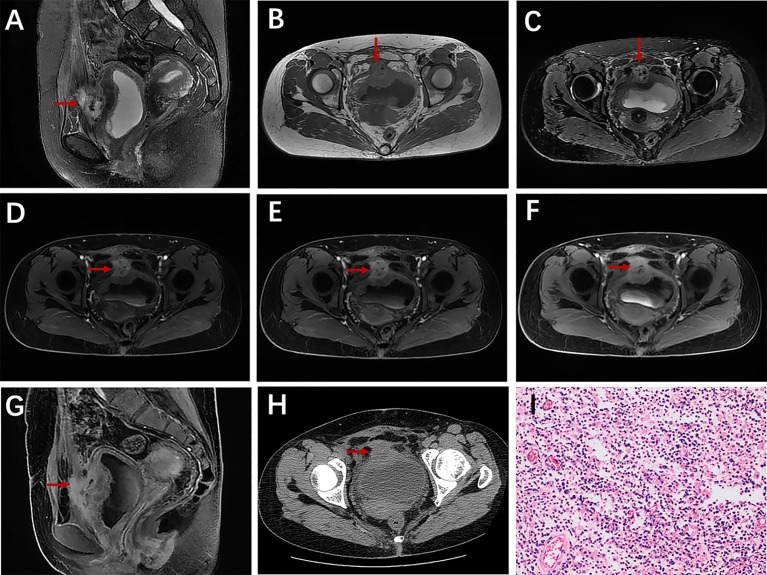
A 38-year-old woman with urachal infection presented with pollakiuria and dysuria. Sagittal T2WI (A) showed a mainly solid mass in the urachal region; iso-intensity on T1WI (B) and T2WI (C), and the adjacent bladder wall thickened obviously; axial dynamic contrast-enhanced LAVA-Flex images and sagittal contrast-enhanced images (G) showed obvious enhancement (D–F); no calcification on axial CT plain scan (H); pathological findings confirmed urachal infection (I).
